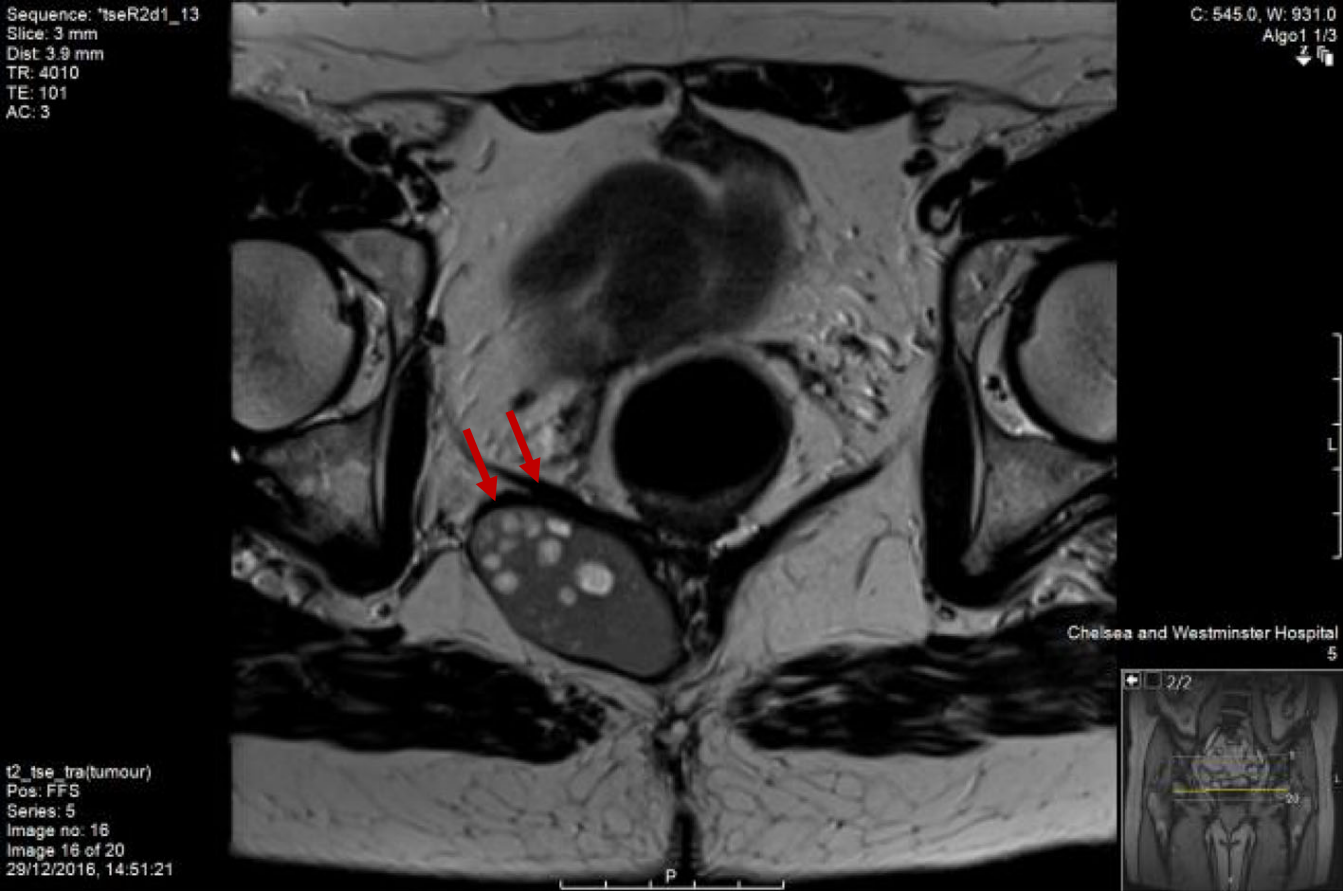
MRI scan image—transverse view
